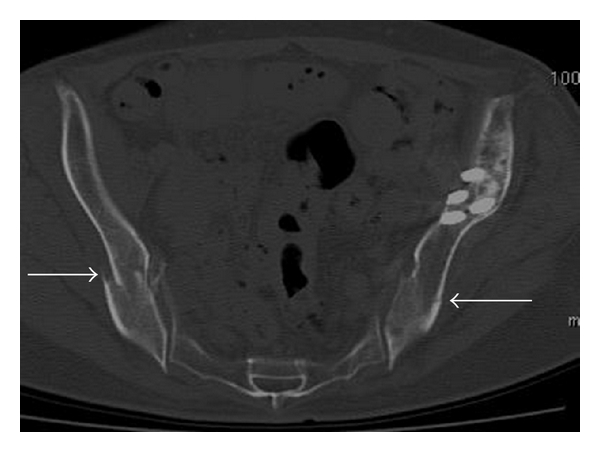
CT image showing pelvis. Arrows indicate fracture site of iliac bone.
